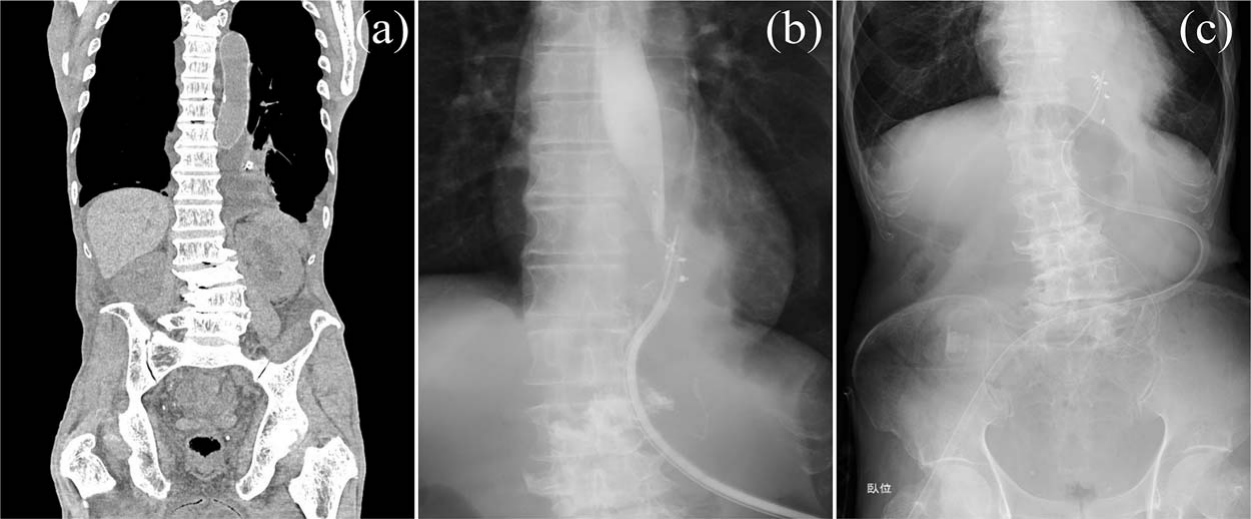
Postoperative imaging and monitoring. (a) Chest and abdominal CT scan demonstrating mediastinal abscess formation. (b) Contrast study through drainage tube revealing communication with the esophagus. (c) Chest radiographs monitoring pulmonary status and drain positioning.
